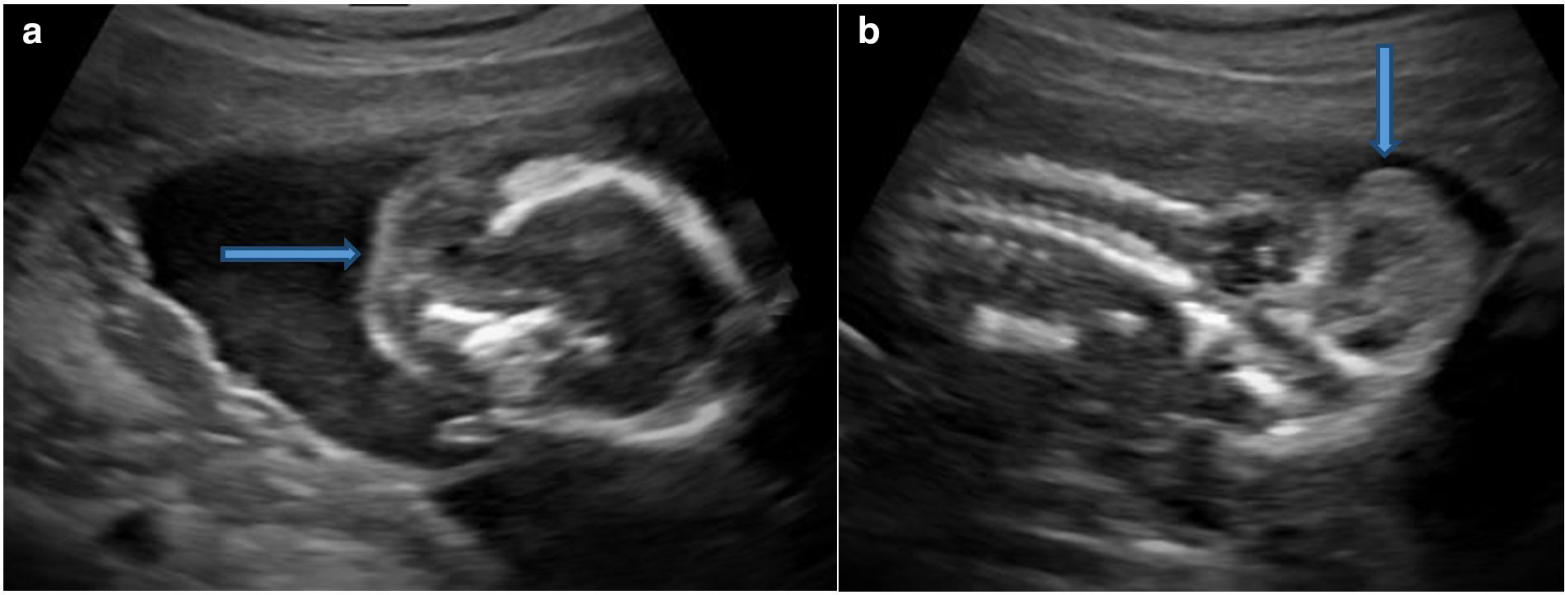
Ultrasound. Axial section (A) and Oblique coronal section (B) of fetal brain showing occipital encephalocele (block arrow).
